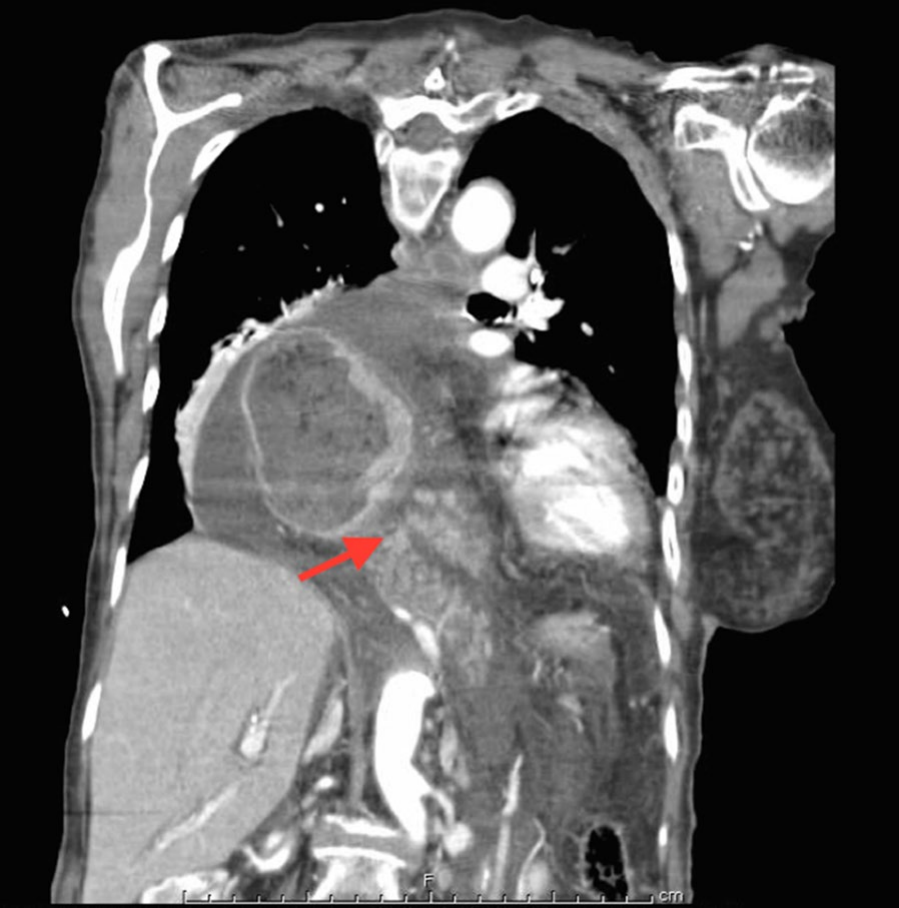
Coronal section: CT scan of the chest and abdomen, which shows the herniation of the pancreas (body and tail) into the pancreas (*arrow*)
